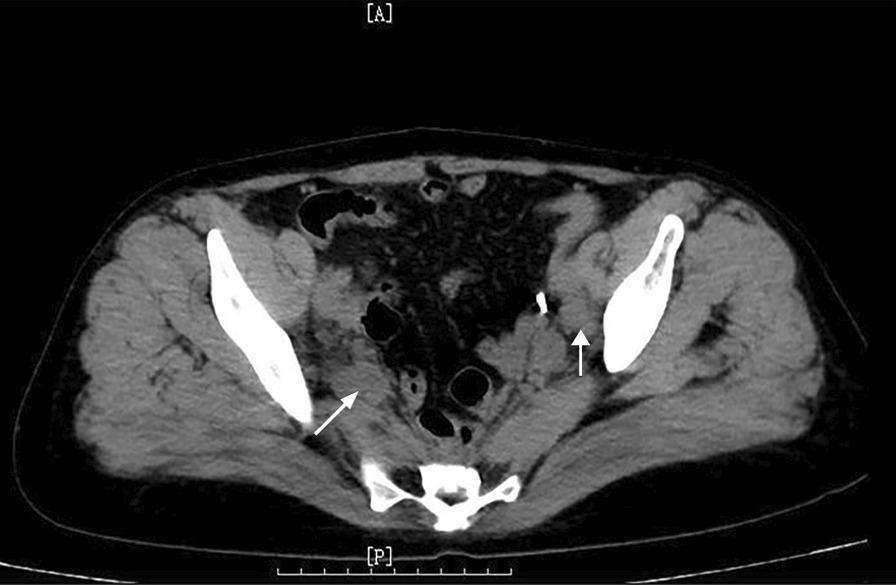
Pelvic CT showed that the lateral lymph nodes were enlarged (arrow) before interventional angiography
